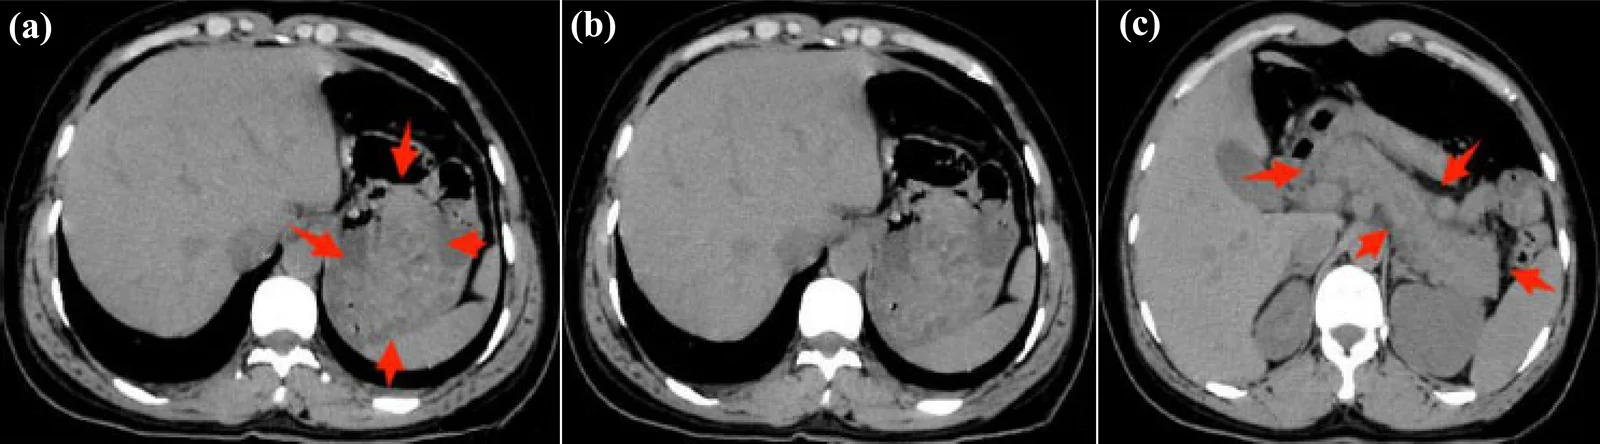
Abdominal CT images showing: A heterogeneous, irregular mass-like density shadow within the stomach, approximately 83 mm x 38 mm x 51 mm (anteroposterior x transverse x craniocaudal), with internal structures resembling curled, ribbon-like objects. The shape is irregular, and the boundaries are poorly defined. Fig. (1c) CT image showing an enlarged pancreas with surrounding exudation.
